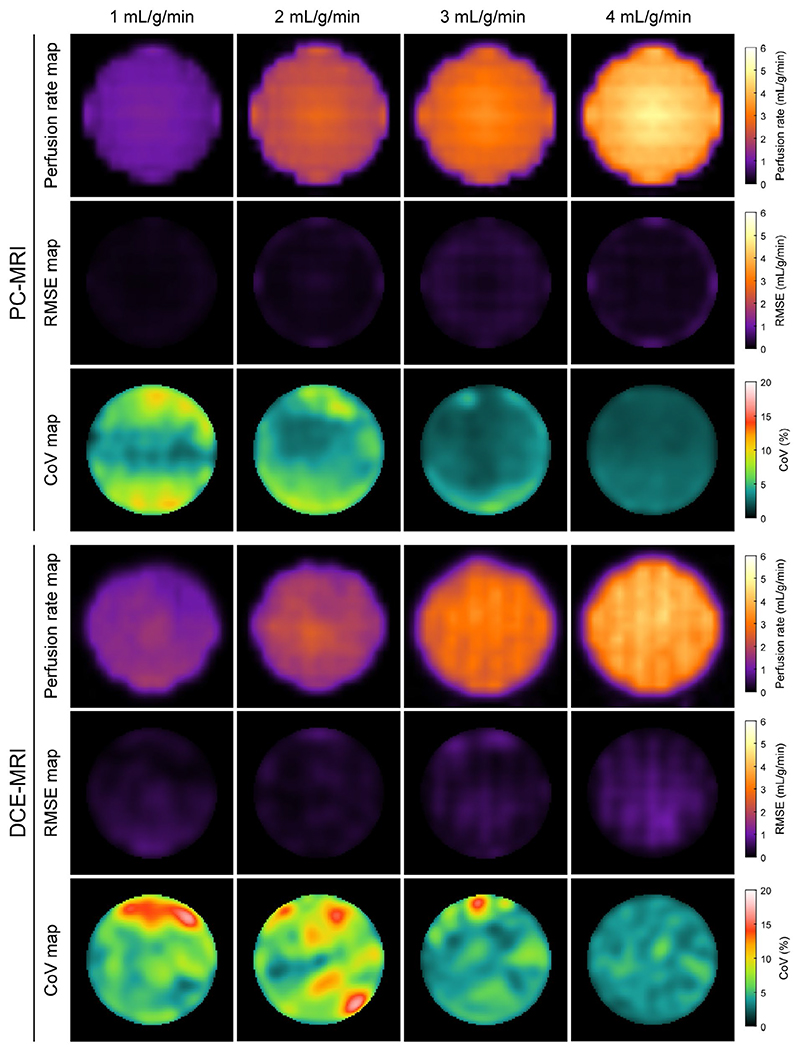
Pixel-wise assessment of perfusion rate by phase contrast (PC)-MRI and dynamic contrast-enhanced (DCE)-MRI. Perfusion rate maps and corresponding root mean square error (RMSE) and coefficient of variation (CoV) maps are shown, assessing the accuracy against reference maps and the repeatability respectively. The surrounding static tissue was cropped out of the maps to enhance visualization. The reference maps were generated using computational fluid dynamics and are provided in Supporting Information Figure S2
